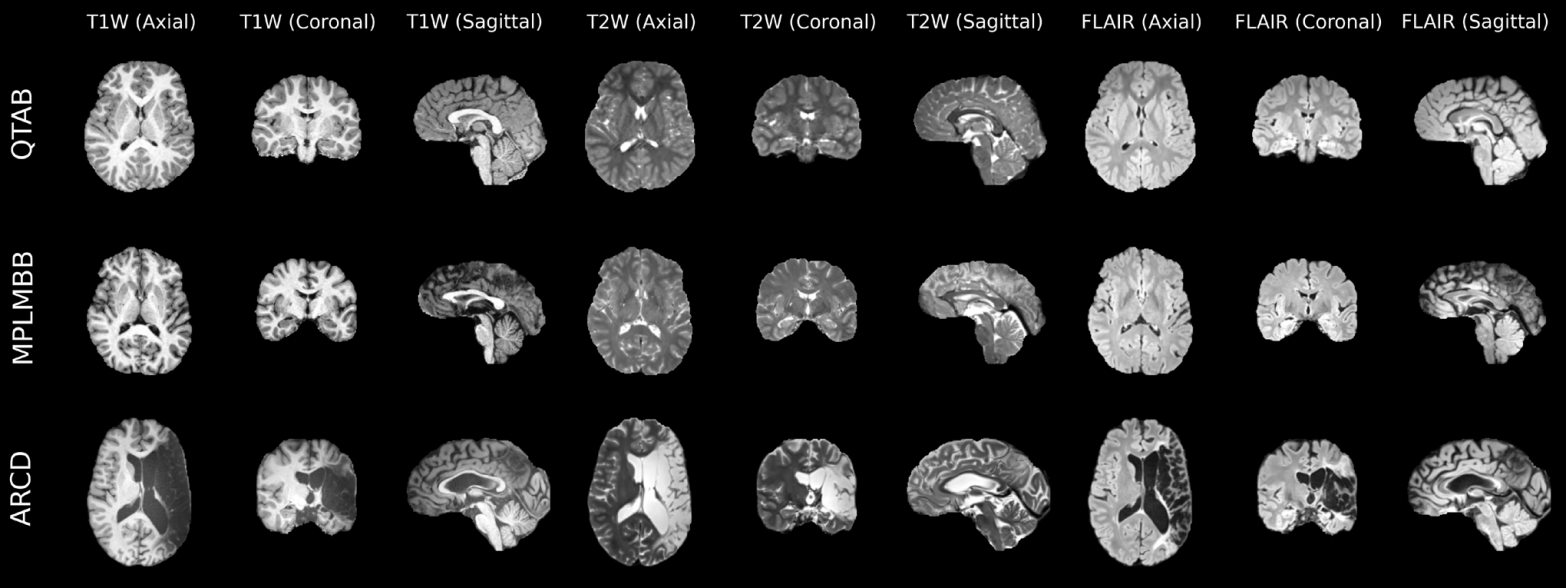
Multimodal MRIs of three subjects from different datasets with each subject having T1w, T2w, and FLAIR modalities. The vertical axis (Y-axis) lists each subject by its source dataset identifiers (detailed in the Supplementary Material), while the horizontal axis shows axial, coronal, and sagittal slices for each modality. Here, the subject from the “QTAB” dataset is a 10-year-old female, the subject from “MPLMBB” is a male aged between 65 and 70 years, and the subject from “ARCD” is a 40-year-old male with a stroke.
